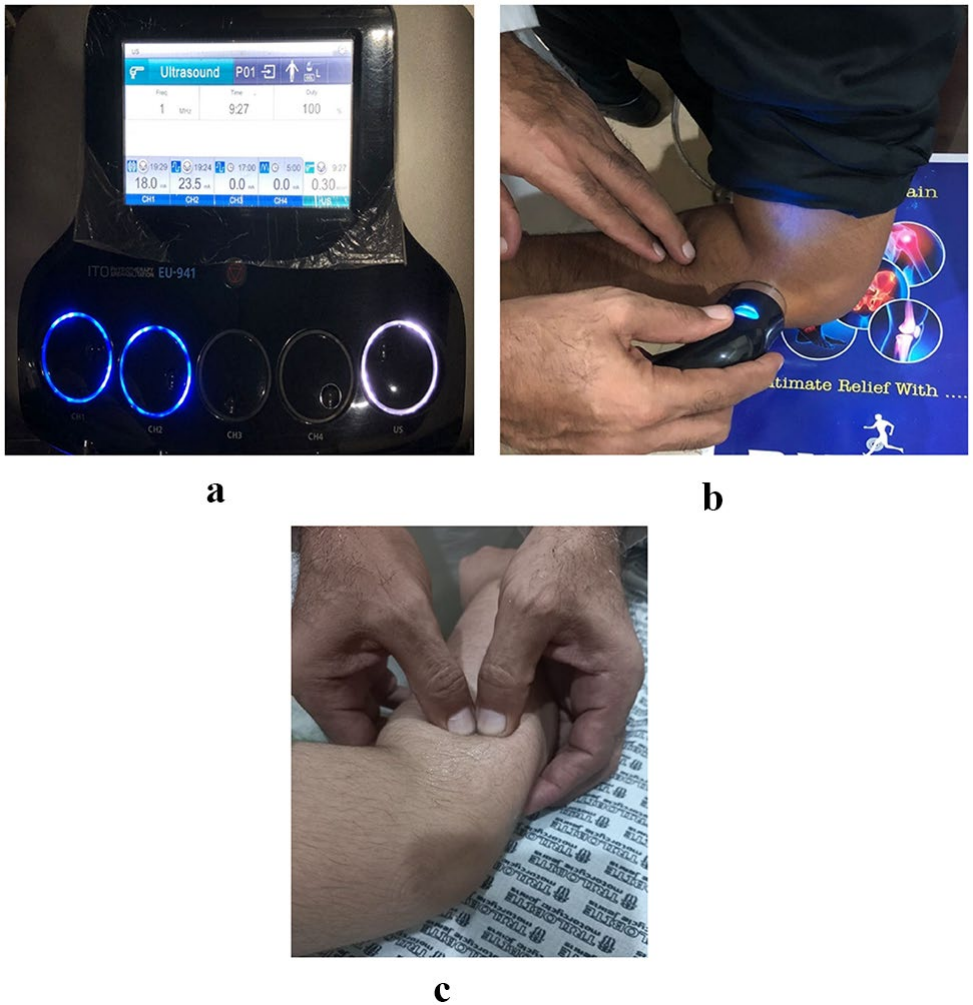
(a) Output settings of the ultrasonic therapy device for a patient with lateral epicondylitis. (b) Application of the ultrasonic therapy for a patient with lateral epicondylitis. (c) Application of the deep tissue friction massage for a patient with lateral epicondylitis.
